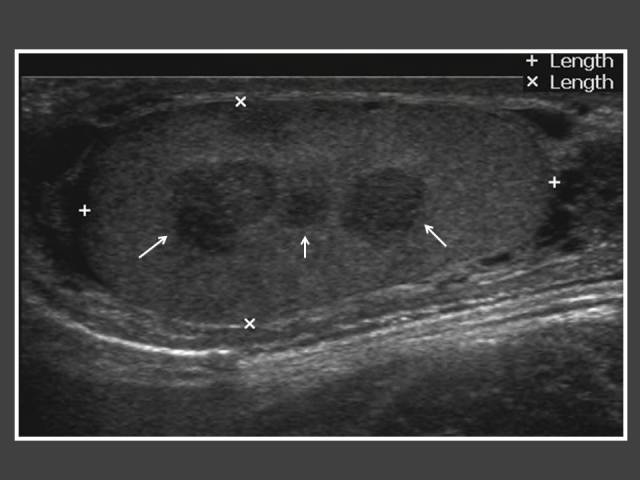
Twelve year-old boy who was diagnosed earlier with congenital adrenal hyperplasia at the age of 2 and was on steroid treatment. An ultrasound of the testis was performed because of resistance to steroid therapy. It shows multiple hypoechoic lesions within the testis (arrows) representing testicular adrenal rest tumour.
